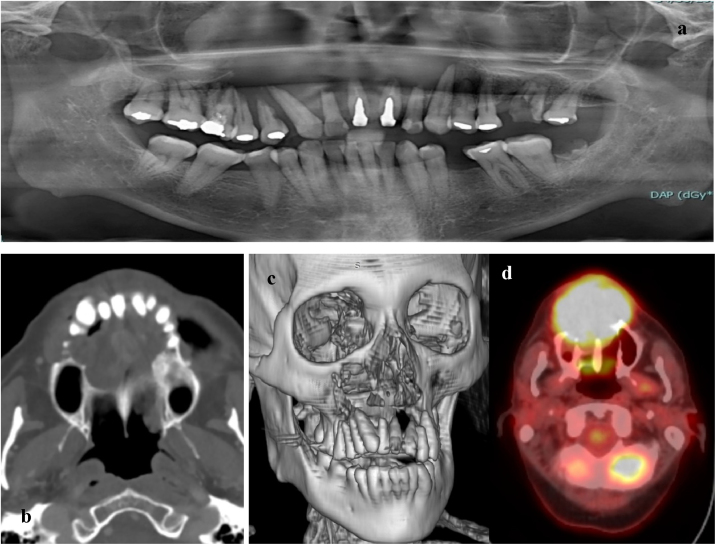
Wide maxillary osteolytic lesion associated with dental root resorption and involvement of the right maxillary sinus: orthopantomography (a), axial CT (b), 3D reconstruction (c) and PET (d) images.
